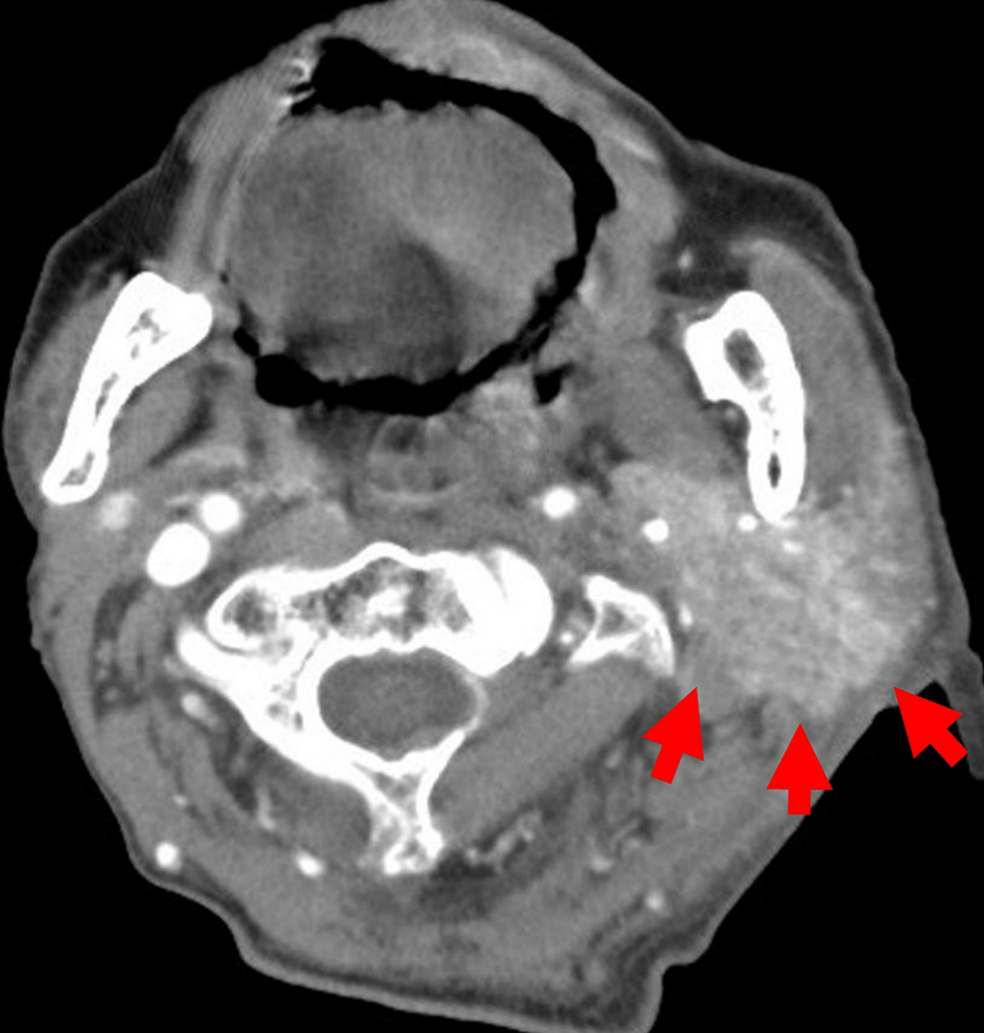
Contrast-enhanced computed tomography (CT) of the neck showing asymmetrical swelling and enhancement of the left parotid gland (arrows).
